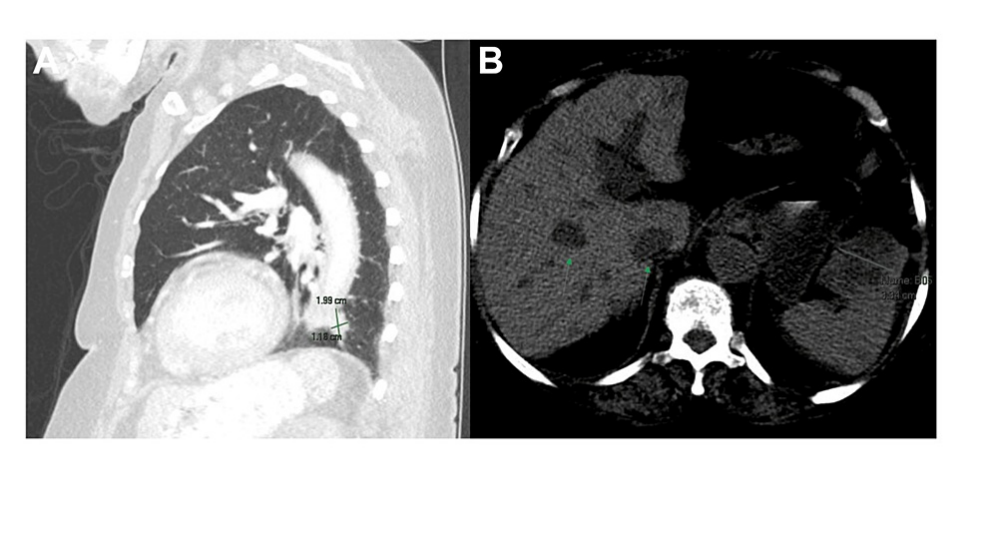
CT scan of chest, abdomen, and pelvis revealed (A) pulmonary mass in the left lower lobe and (B) hepatic masses.
